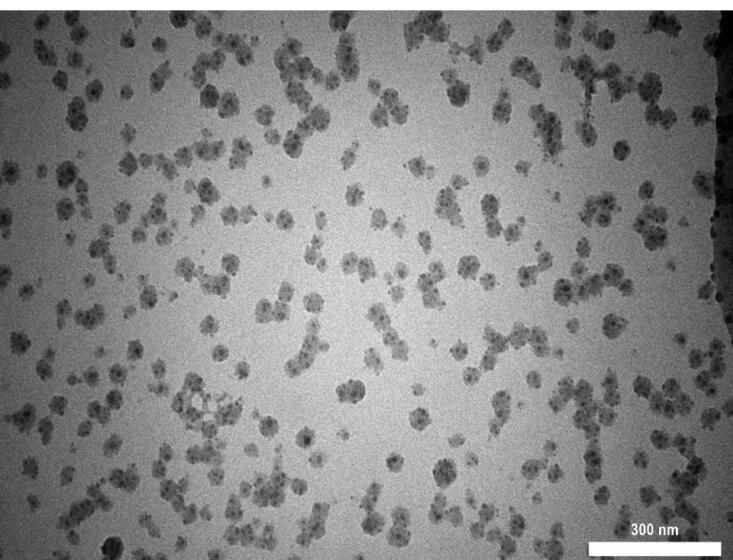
###  Energy dispersive X-ray
